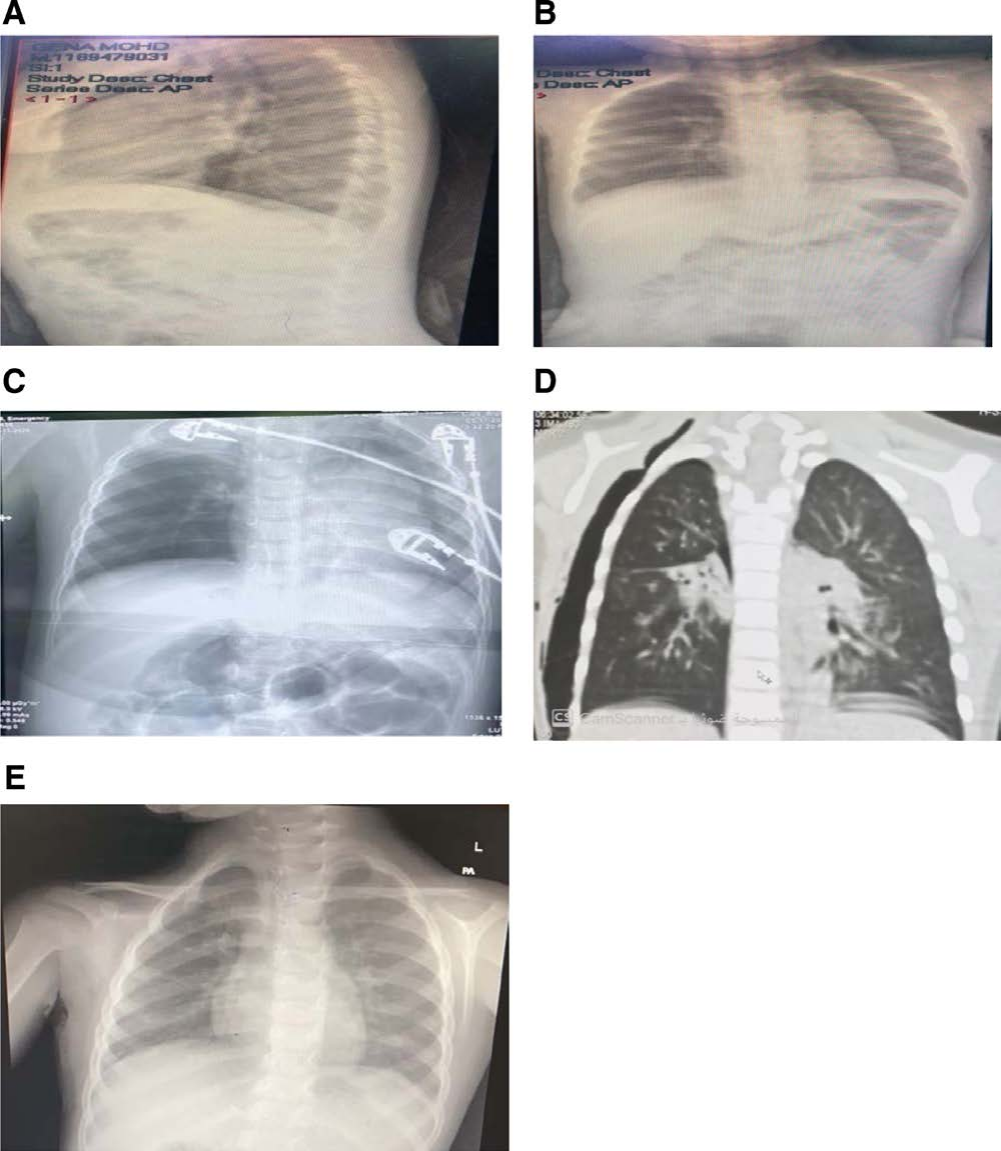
Chest radiography and CT scan were performed for case 3 before, during, and after FB removal. (A) Preoperative lateral chest radiograph. (B) Preoperative AP chest radiograph. (C) Intraoperative chest radiograph showing right lung collapse and pneumothorax. (D) Coronal view of the chest CT showing subcutaneous emphysema. (E) Postoperative and post-ICD removal chest radiograph. AP = anteroposterior, CT = computed tomography, ER = emergency room, FB = foreign body, ICD = Intercostal drainage tube.
